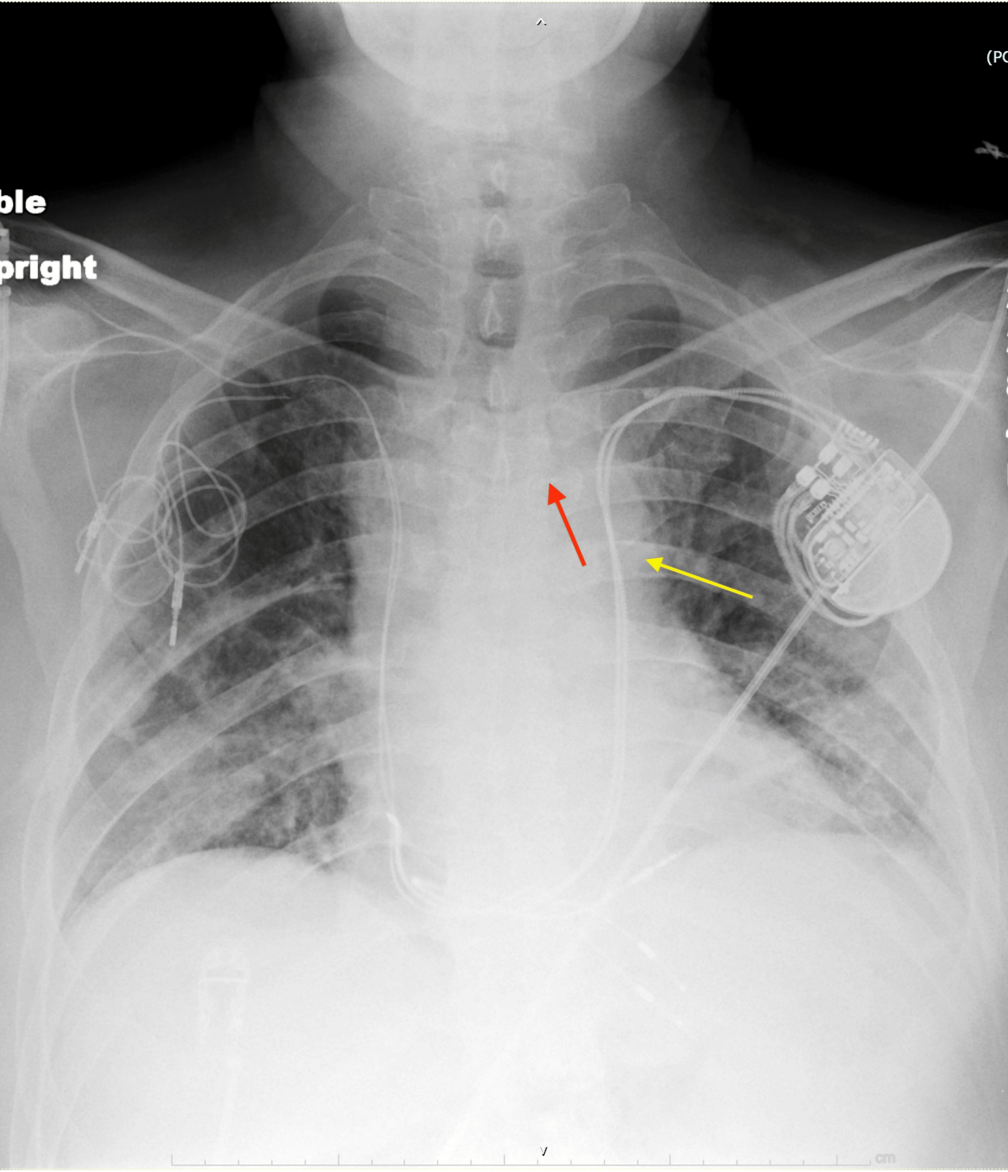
Chest X-ray showing the newly implanted left-sided lead passing through the PLSVC. The left-sided dual chamber device with right atrial and ventricular leads passing through the left brachiocephalic vein was removed (red arrow). A new dual chamber device with right atrial and ventricular leads was implanted through the PLSVC (yellow arrow). PLSVC: persistent left superior vena cava
